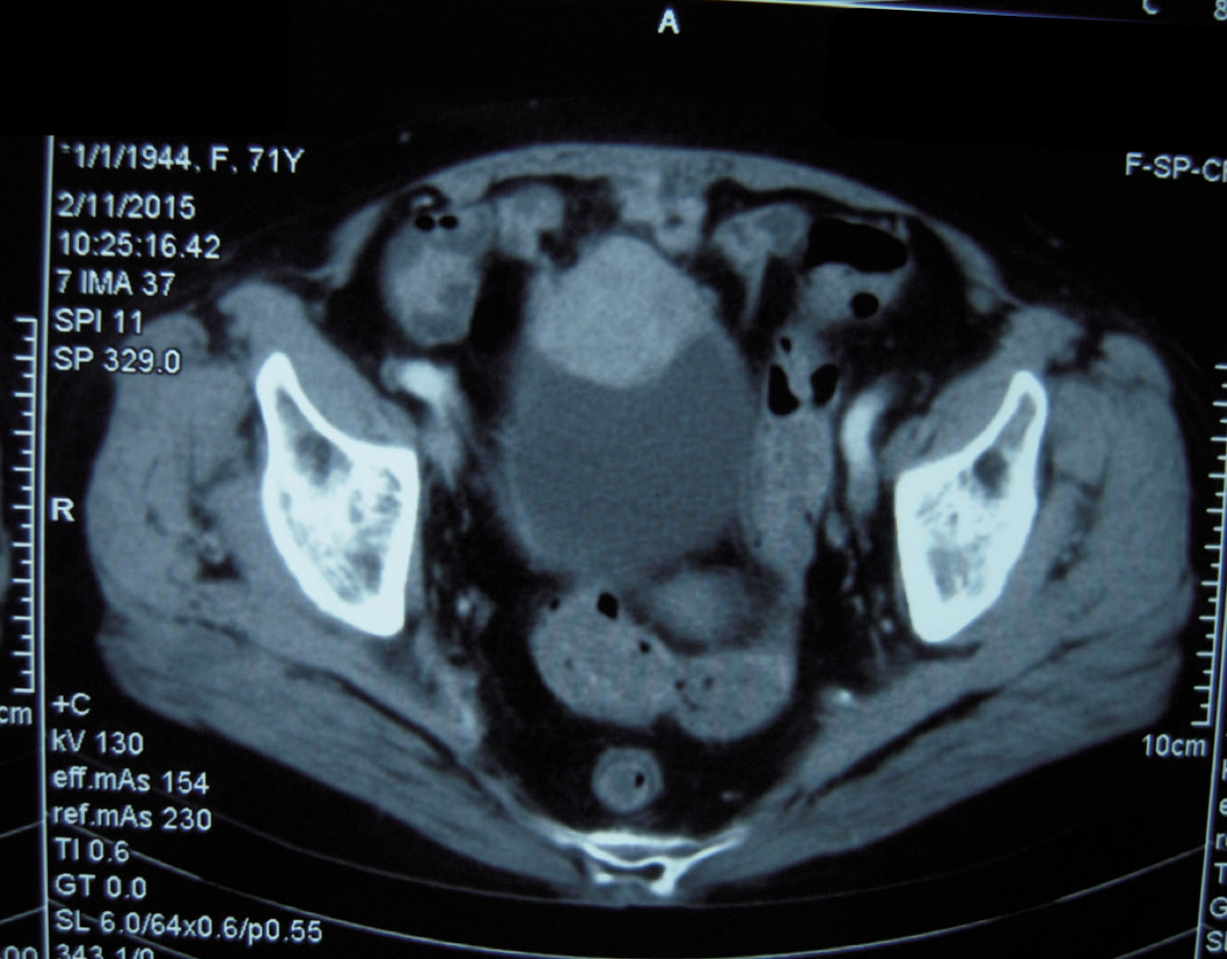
Abdominal computed tomography showed an irregular mass on the anterior wall of the bladder, which involved all layers of the bladder.
